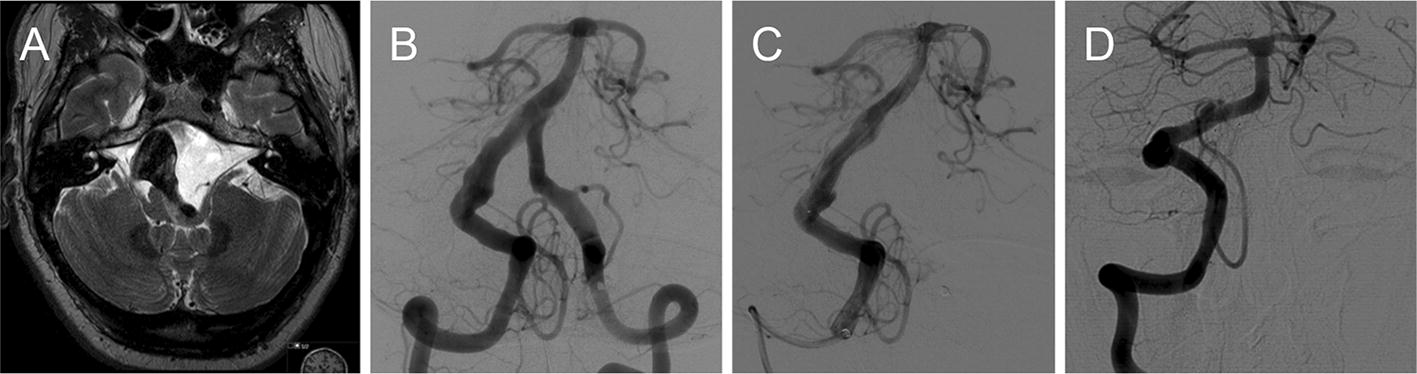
A T2-weighted MRI (magnetic resonance imaging) showing the large, partially thrombosed fusiform aneurysm of the basilar artery with mass effect and edema of the brainstem; B preinterventional DSA (digital subtraction angiography) run showing the partially thrombosed aneurysm as well as the dysplastic V4 segments of both vertebral arteries; C DSA run after placement of three flow-diverter stents in the basilar artery and right vertebral artery. Additionally the left vertebral artery was endovascularly occluded. D DSA control 1 year after the procedure shows a complete occlusion of the aneurysm with a sufficient restitution of the vessel anatomy of the treated V4 segment of the right vertebral artery
